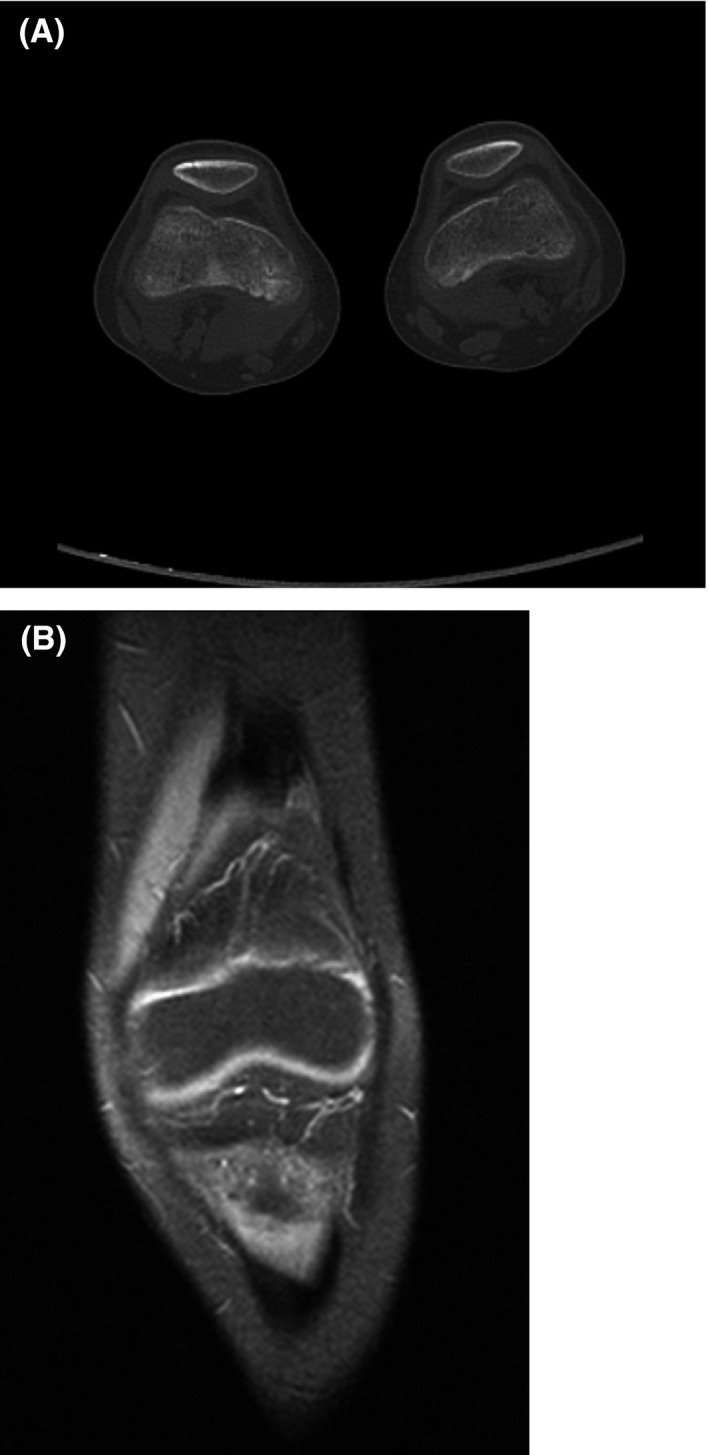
A‐B: Our CT and MRI examination with normal findings
